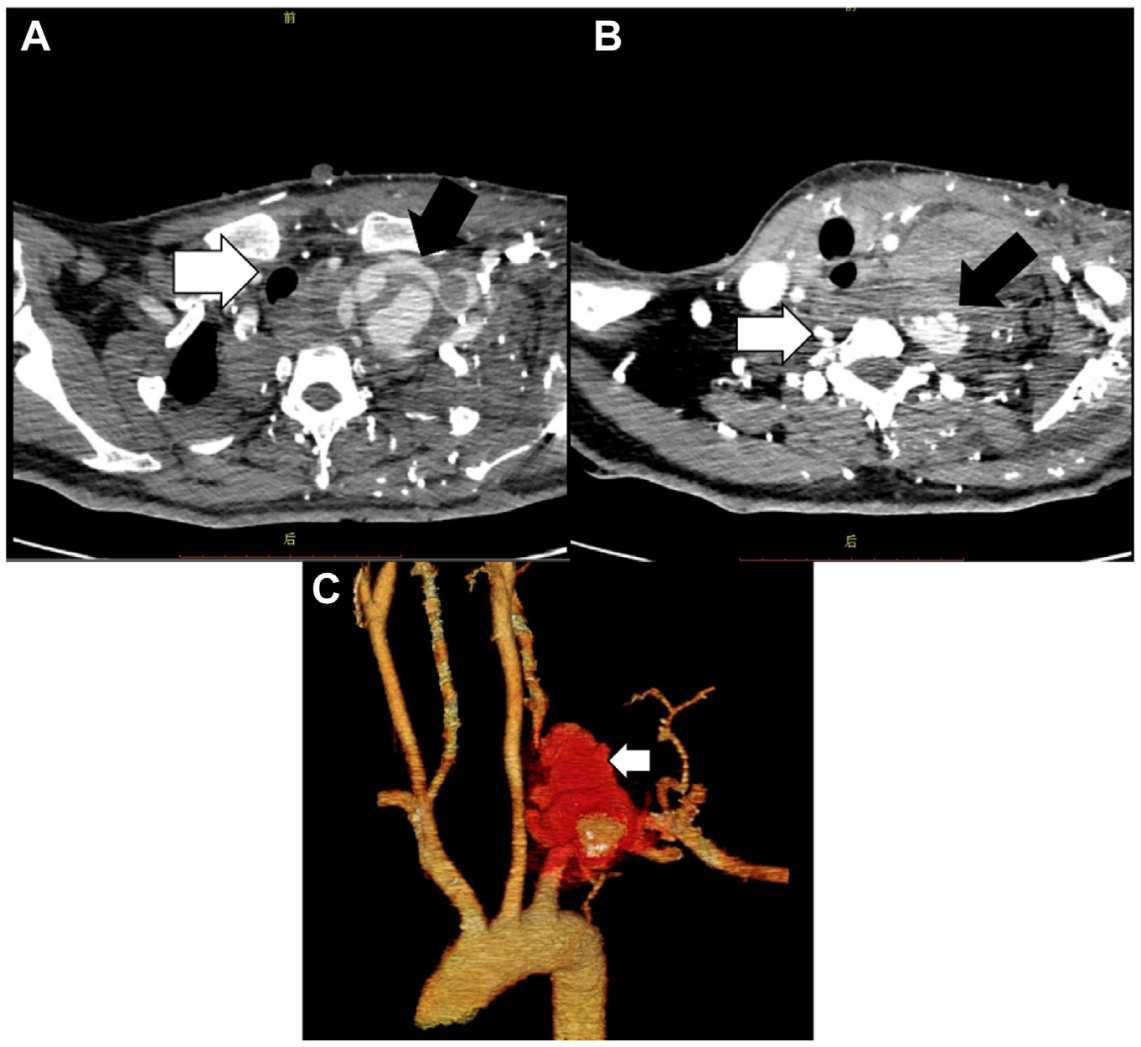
Computed tomography arteriography (CTA) images of case 1. (A) A large hematoma (*black arrows*) can be observed in the patient's thorax, the mediastinum is broadened, and the trachea was deviated to right (*white arrow*). (B) Suspicious rupture of the left vertebral artery. Another hematoma can be seen near the left vertebral artery (*black arrow*), whereas the right vertebral artery is normal (*white arrow*). (C) Three-dimensional reconstructed image showing a massive hematoma (*white arrow*) surrounding the left vertebral artery and the left subclavian artery (LSA).
